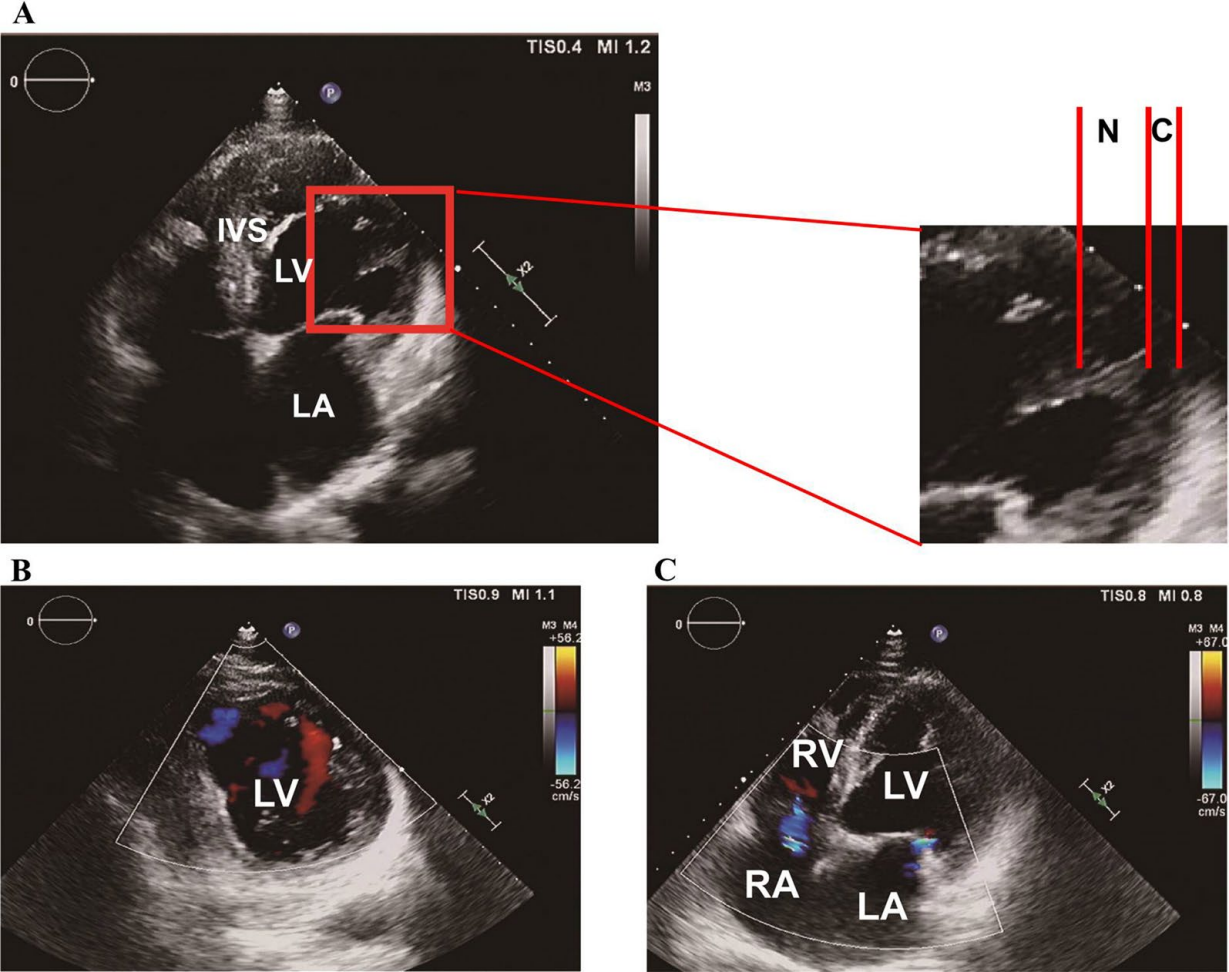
Echocardiographic characteristics of the proband II: 1. A To quantify the extent of noncompaction at the site of maximal wall thickness. The end-systolic ratio of noncompacted to compacted thickness was determined. The two layers were best visualized at end-systole as shown in this long-axis view (N, non-compacted layer; C, compacted layer). B, C Color Doppler study showed typical forward blood flow from the ventricular cavity into the deep spaces between the prominent trabeculations during diastole (in B represented by a red signal). Mild regurgitation could be seen in the mitral and tricuspid valves (C)
